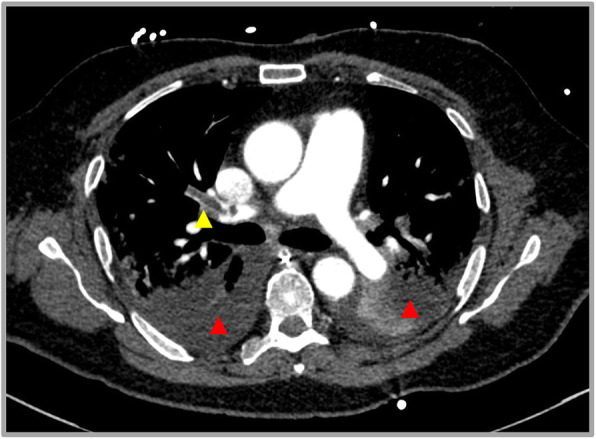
Computed tomography (CT) of chest showing a filling defect in the right upper labor pulmonary artery extending into the segmental and subsegmental pulmonary branches consistent with an acute pulmonary embolism (yellow arrowhead). Patchy infiltrates are indicated by the red arrowhead
